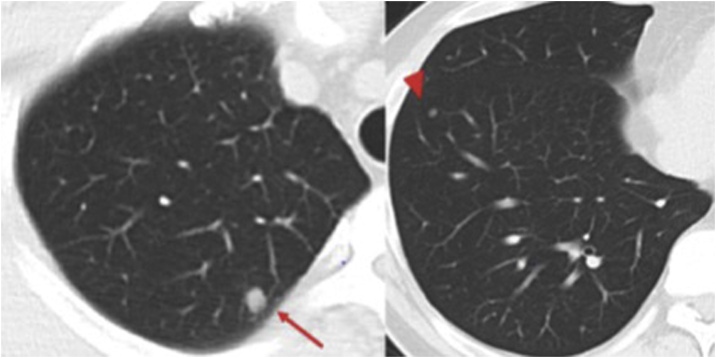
Chest computed tomography showing pulmonary nodules in the right upper (arrow) and lower lobes (arrowhead).
